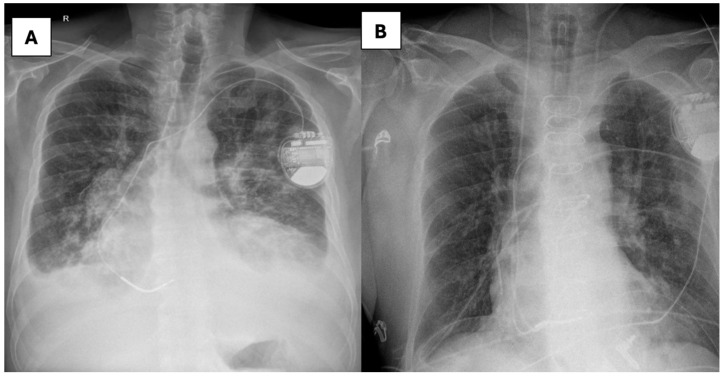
Chest radiographs obtained before and after surgical ventricular reconstruction. (A) Preoperative chest radiograph demonstrating marked cardiomegaly, bilateral pleural effusions, and pulmonary vascular/interstitial congestion consistent with advanced congestive heart failure. An implantable cardioverter-defibrillator (ICD) is visible in the left pectoral region. (B) Early postoperative chest radiograph showing a reduction in cardiac silhouette size and improvement of pulmonary congestion following surgical treatment.
